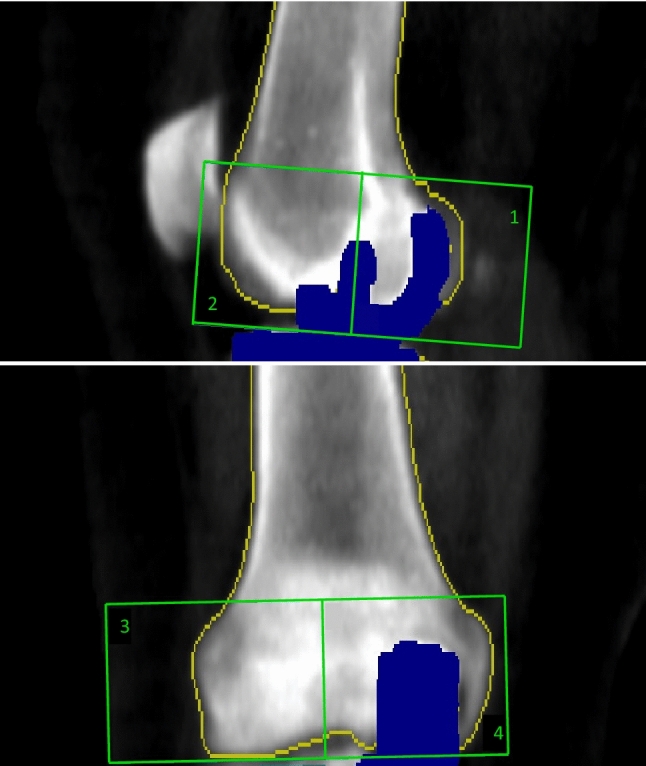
Dual-energy X-ray absorptiometry regions of interest for the evaluation of bone mineral density. The blue area marks the implant and is removed from the BMD measurement. The yellow line marks the bone border for BMD measurement. On LA scans, the division between ROI1 and ROI2 was done in the center of the large peg. On AP scans, the division between ROI3 and ROI4 was done in the intercondylar area
